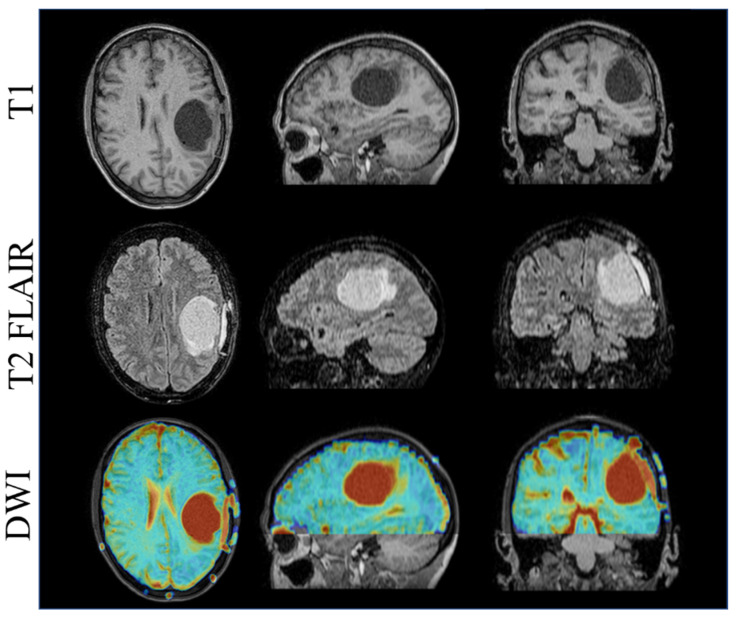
T1 (Top), T2 FLAIR (Center), and DWI (Bottom)-imaging 1.5T MR-linac.
